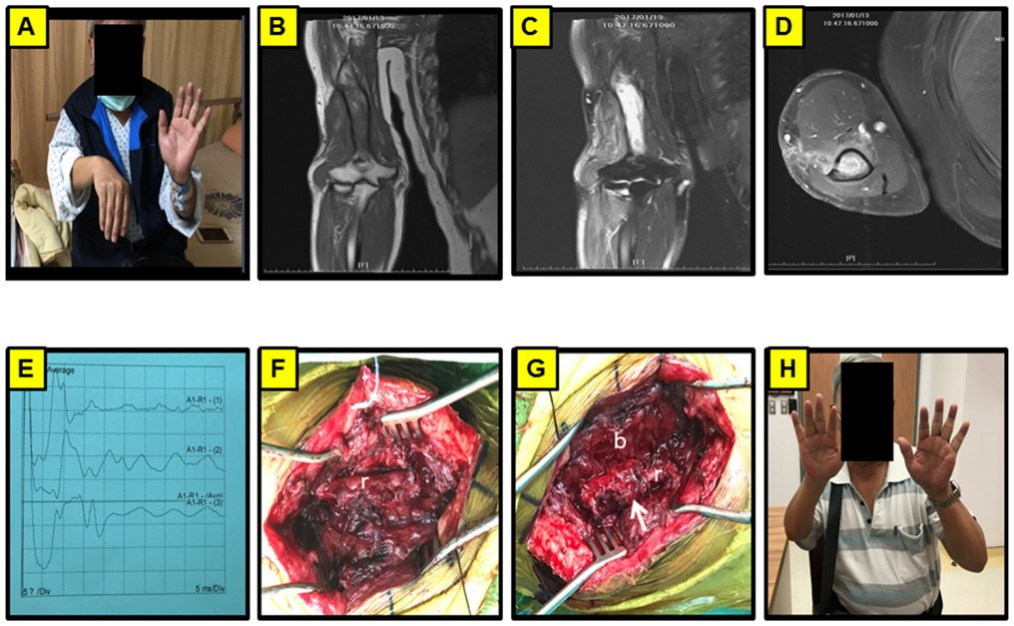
Representation of a right humerus fracture after ORIF complicated with osteomyelitis. (A) 68-year-old male presented with right wrist drop for 6 months after humerus fracture after operation. (B) MRI of right upper limb in T1W1 in coronal view. (C) MRI of right upper limb in T1W1 with contrast administration in coronal view. (D) MRI of right upper limb in T1W1 with contrast administration in axial view. (E) Intraoperative recording of CMAP stimulated from the distal to proximal end of the nerve. (F) The photography showed intact nerve but with severe fibrotic change. (G) Rotation of the muscle flap to wrap the injured region. (H) The patient showed favorable neurological outcome with restoration of wrist function 2 months after the operation. b: biceps muscle; r: radial nerve.
